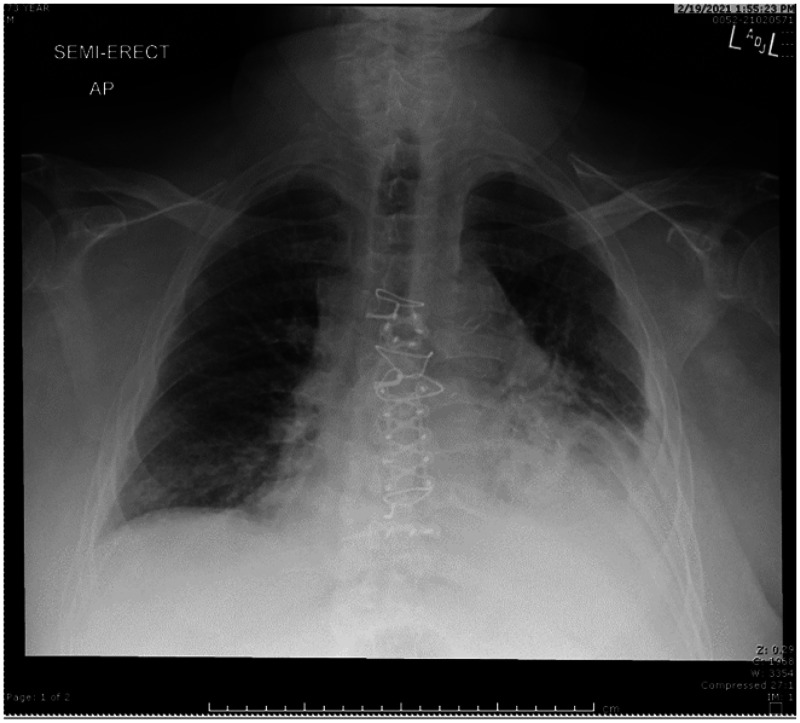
Chest X-ray obtained upon initial presentation. Notable for left lower lobar consolidation with associated pleural effusion, and atelectasis.
